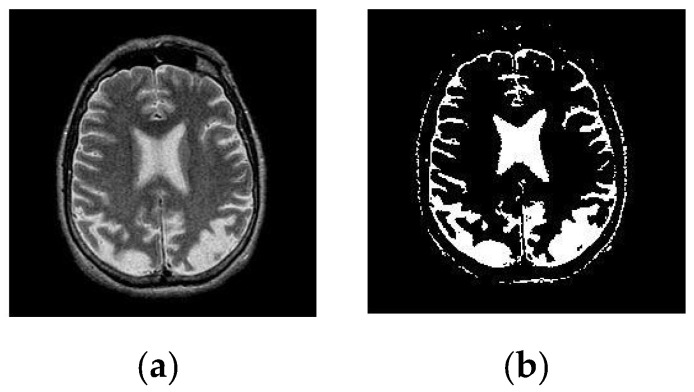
Here, image (a) is the input T-1 brain MRI image and image (b) is the segmented T-1 image. A segmented image classifies it as a Benign Tumor.
